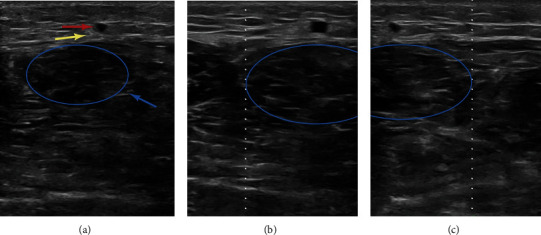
A male patient, 28 years old, with an Achilles tendon rupture in a football match. The pictures show the anatomical structure on the plane of the proximal end of the tendon (9 cm from the proximal end of the broken end of the Achilles tendon) examined by ultrasound. In this plane, the small saphenous vein and sural nerve are located directly above the Achilles tendon. (a) The blue arrow shows tendons; the red arrow shows the small saphenous vein; the yellow arrow shows the sural nerve. (b) The dotted line shows the lateral edge of the Achilles tendon. (c) The dotted line shows the medial edge of the Achilles tendon.
